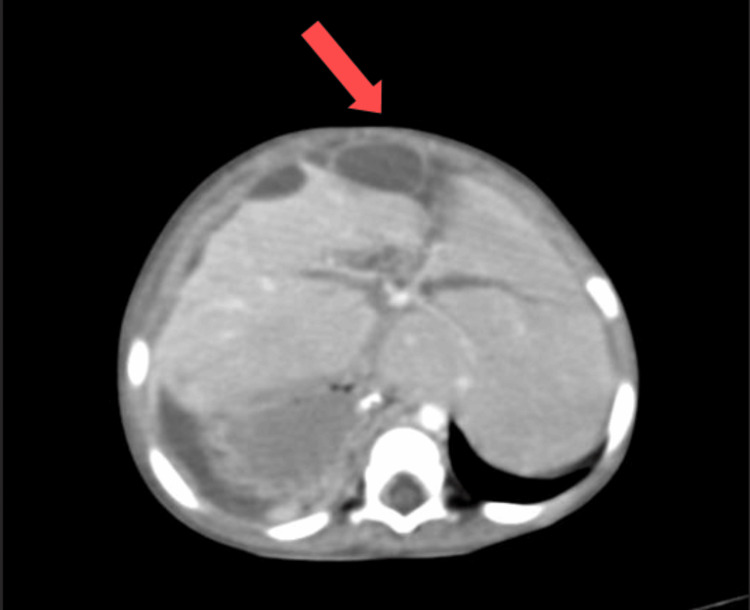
Axial view of abdominal CECT showing subcapsular hypodense collections (red arrow). CECT: contrast-enhanced computed tomography
